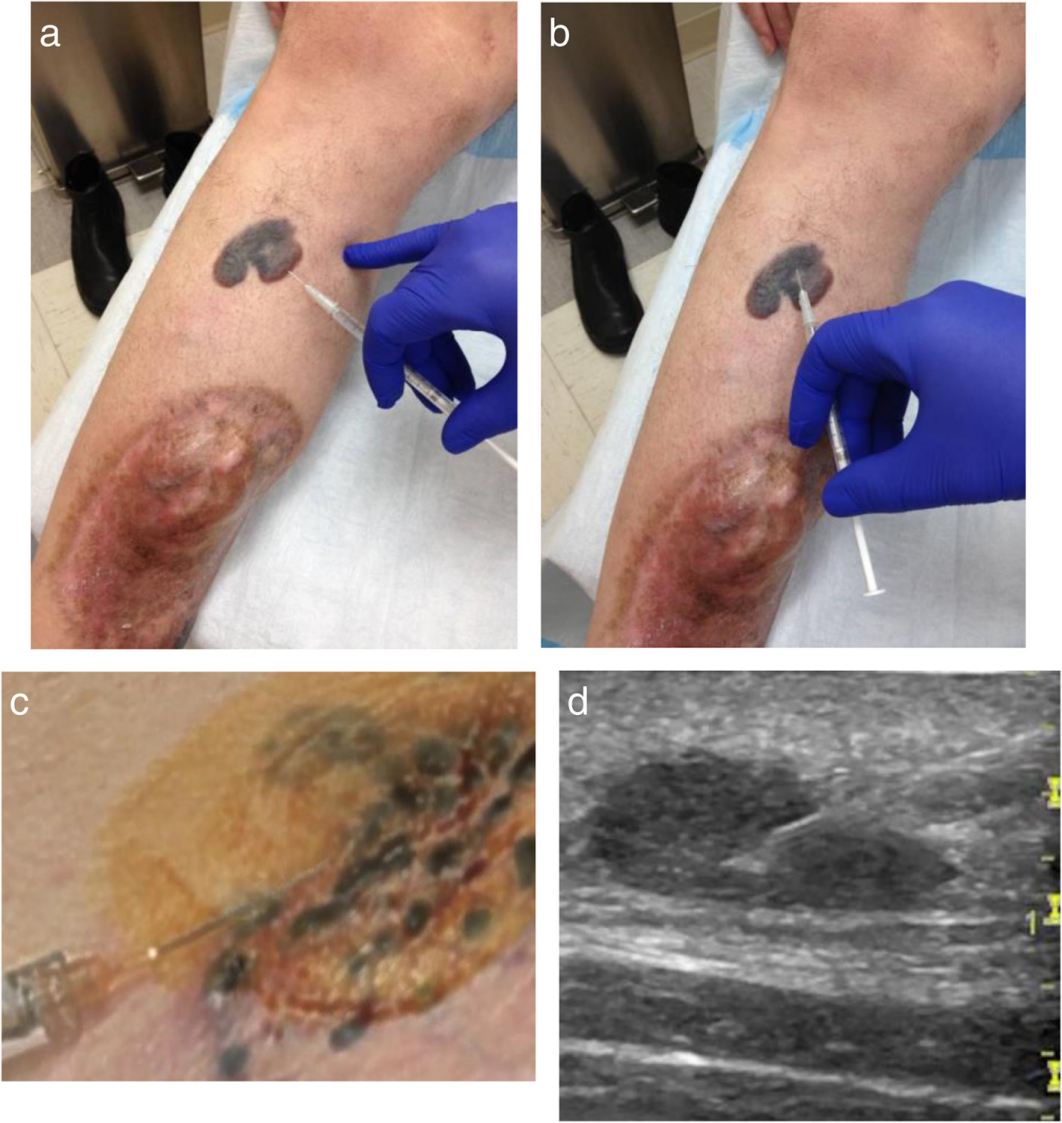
T-VEC administration. a Fan technique. Enter at a single insertion point as far as the needle allows within the lesion to achieve even and complete dispersion. Inject within the lesion by pulling the needle back without exiting the lesion. Redirect the needle as many times as necessary in the shape of a fan while injecting the remainder of the dose of TVEC. Continue until full administration of vial. b Four quadrant technique. Mark the lesion in four different quadrants 2–3 mm apart. Enter one of the marked areas with the needle and inject virus. Exit the quadrant and move on to different area and repeat in all four quadrants until complete administration of dose. c Clustered dermal metastases. When lesions are clustered together, they can be measured and injected as a single lesion. d Ultrasound. Ultrasound assistance can be used for lesions that are difficult to identify
